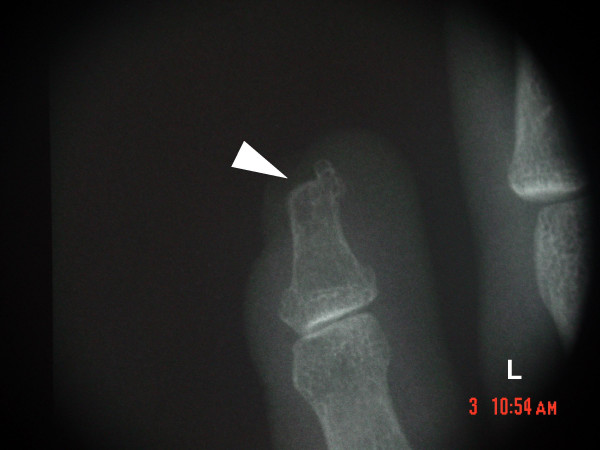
Left hand radiograph showing characteristic abnormal fifth distal phalanx (arrow) in 46,XX del(4)(q32). The defect was bilateral.
